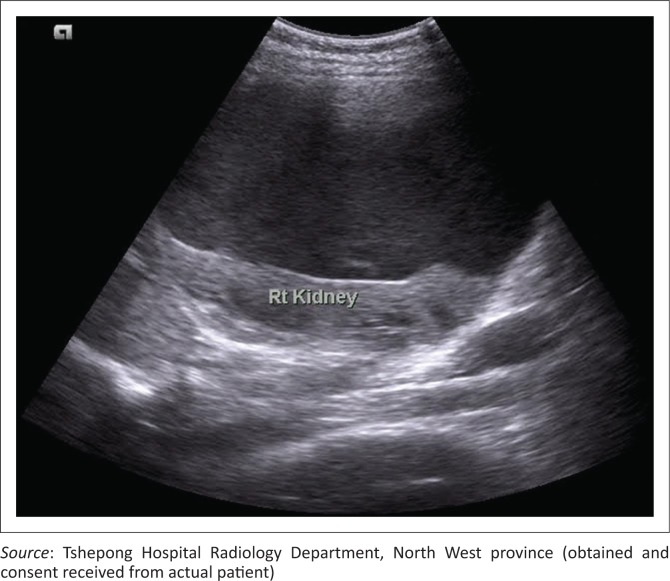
Sagittal ultrasound image of the right kidney depicting a large perirenal hypoechoic fluid collection with hyperechoic internal echoes compatible with complex fluid. Associated medial displacement and compression of the right kidney. Similar findings were seen on the contralateral side (not shown)
